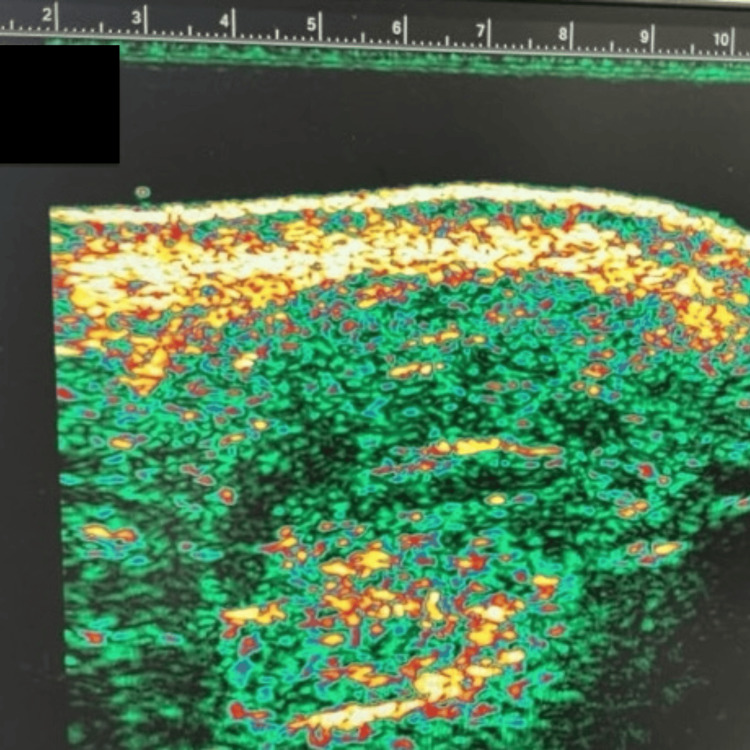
High-resolution dermal ultrasound obtained after completion of 20 treatment fractions over six weeks, with an additional six-week recovery period, demonstrating no detectable residual tumor.
